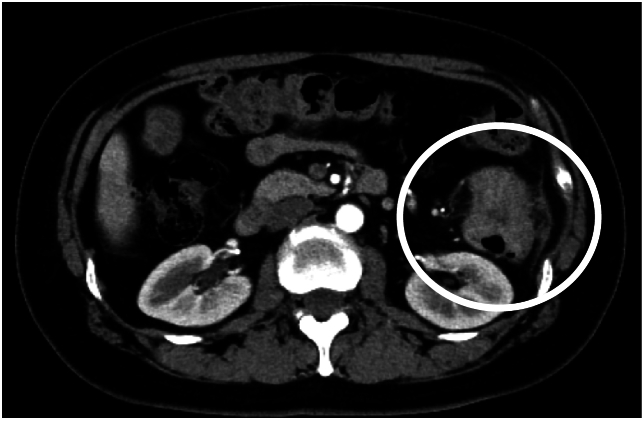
Imaging findings for the diagnosis of colorectal cancer. Computed tomography (CT) shows a 5 cm mass in the splenic curvature of the descending colon (white circles).
